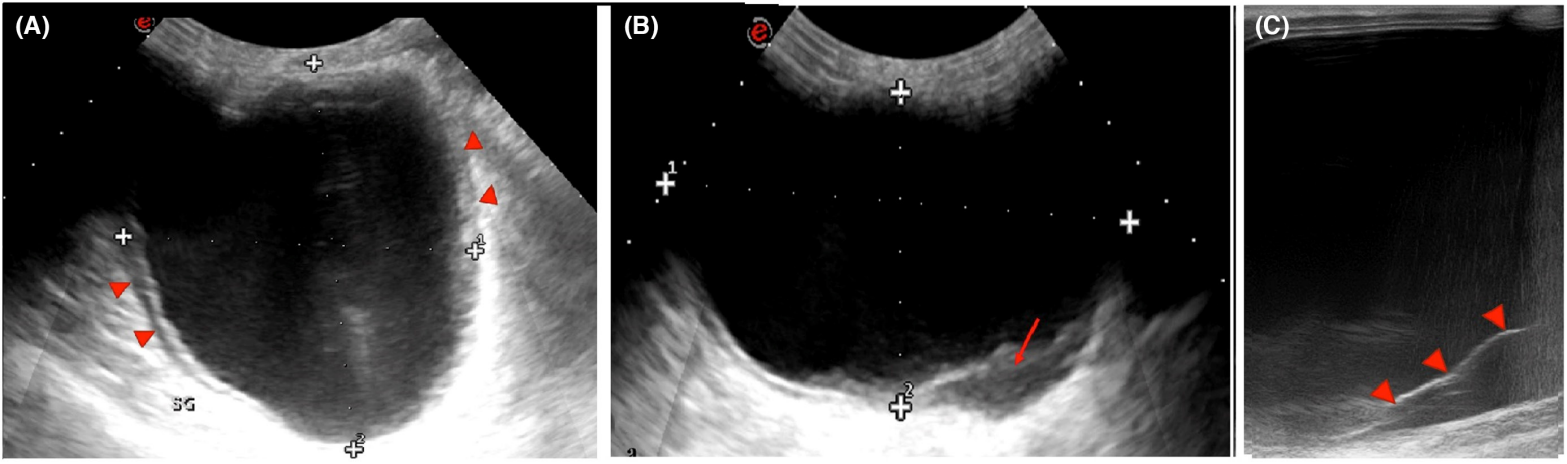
Ultrasound of the left breast shows: *(A)* a unilocular anechoic cystic lesion, with a thickened wall(arrowheads); *(B)* an echogenic sediment consistent with hydatid sand (arrow); and *(C)* a detached posterior membrane(arrowheads)
